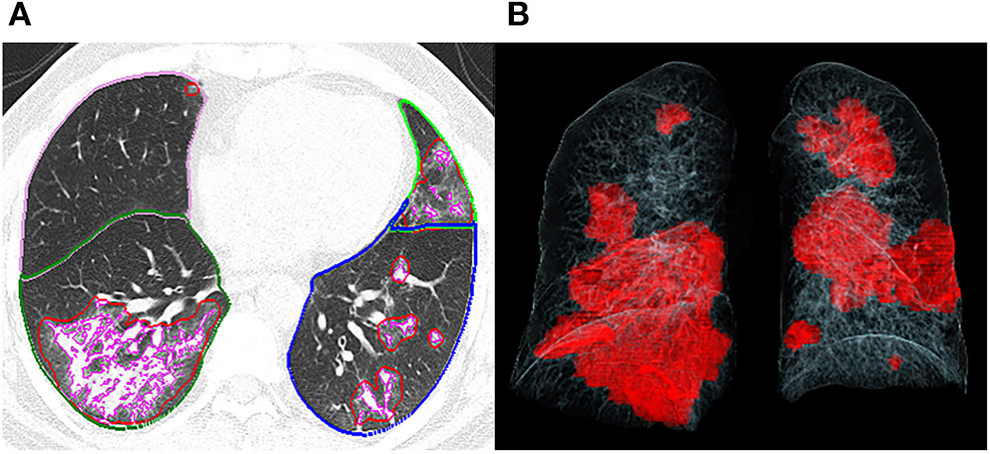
Representative postprocessing result of chest CT scan performed with Siemens CT Pneumonia Analysis. A 56-year-old male with COVID-19 (Opacity score = 5, volume of opacity = 671.6 ml, percentage of opacity = 15.1%). (A) Axis plane of chest CT shows bilateral ground glass opacities. (B) 3D-visualization of CT volume rendering technique shows the diffuse extent of opacities.
